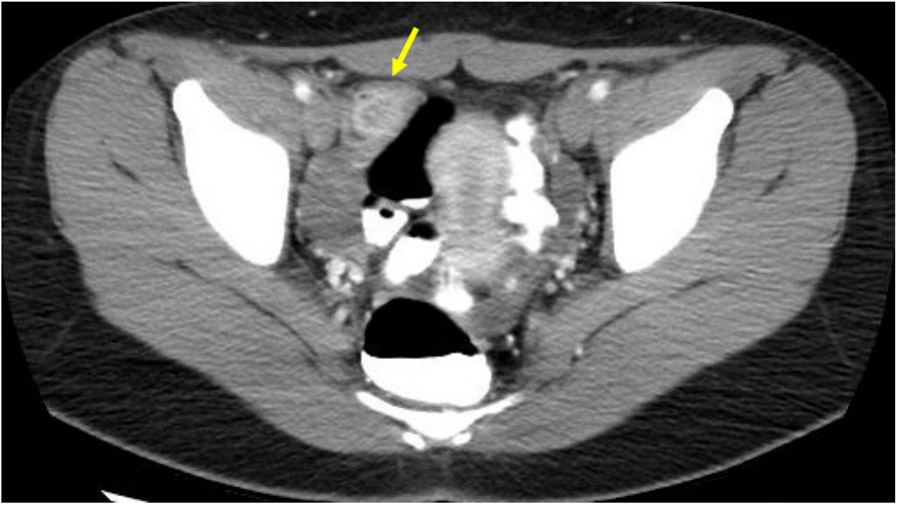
Contrast-enhanced CT images showing a tumor with a contrasting effect in the ileum (*arrow*)
